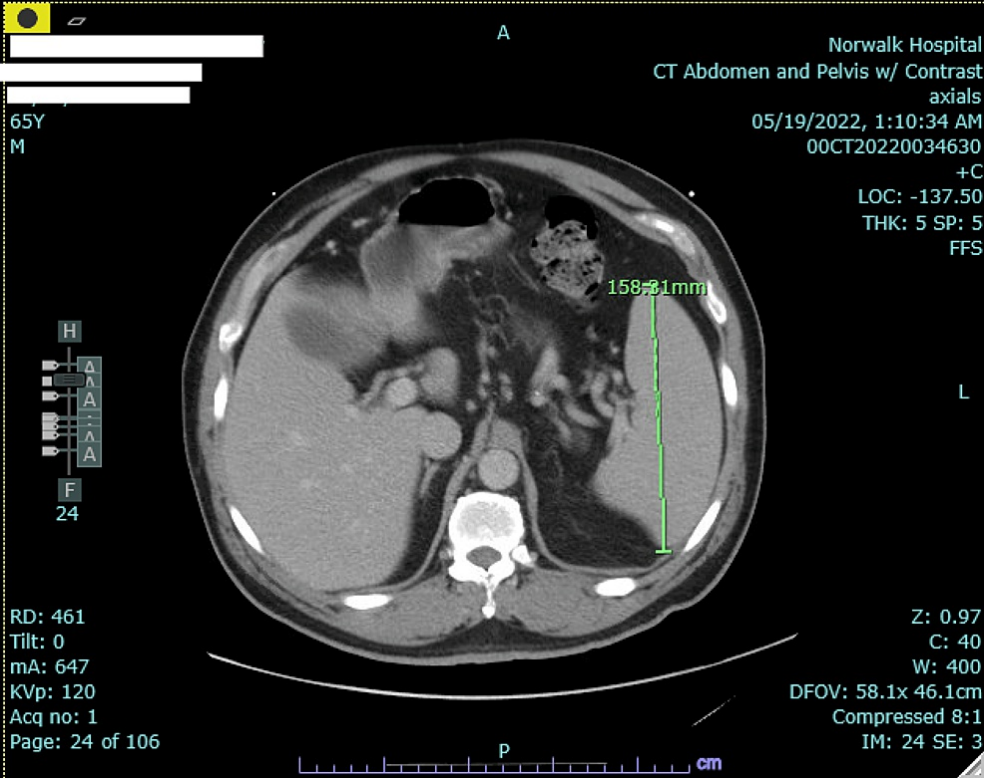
CT scan of the abdomen and pelvis with contrast shows splenomegaly measuring approximately 158.31 mm CT: computed tomography
